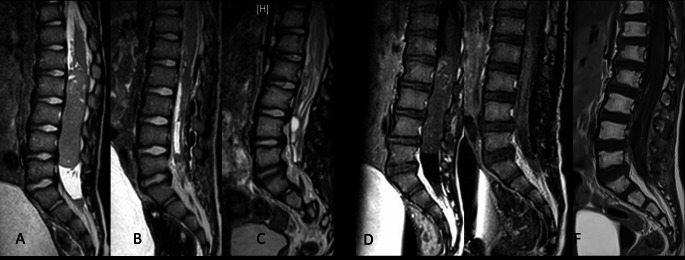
Preoperative (A, D), postoperative (B, E), and one-year follow-up (C, F) MRI images, both sagittal T2 and with contrast, show a spinal ETMR located in the lumbar spine of a 28-months-old child (ID_04). This child experienced severe pain, controllable only with morphine, and acute paraplegia. An urgent laminotomy from L1 to L5 and tumor resection were performed, resulting in total removal of the tumor. This was followed by multimodal adjuvant therapy
